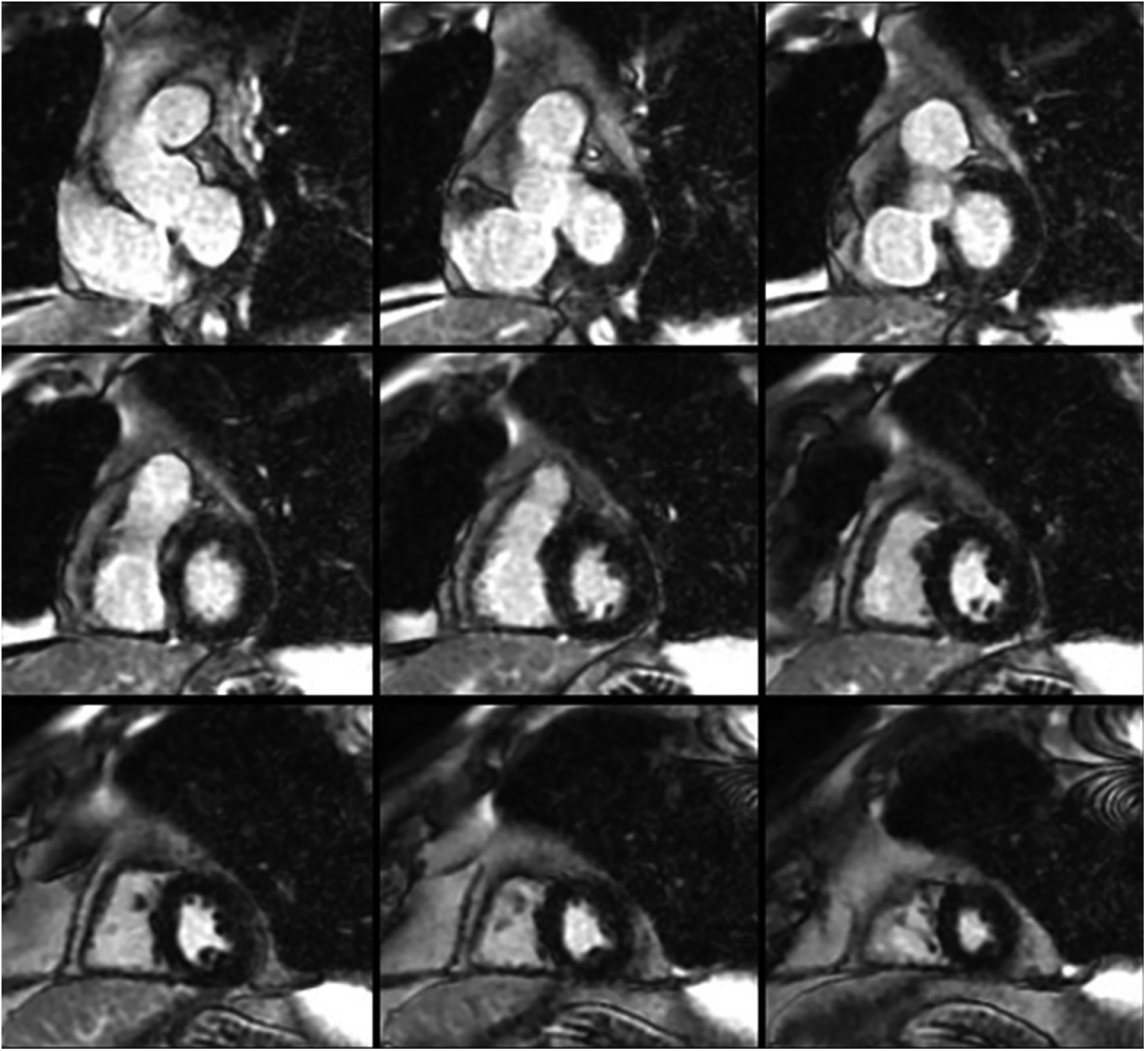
Short-axis cardiac magnetic resonance images demonstrating normal biventricular size and no evidence of late gadolinium enhancement.
